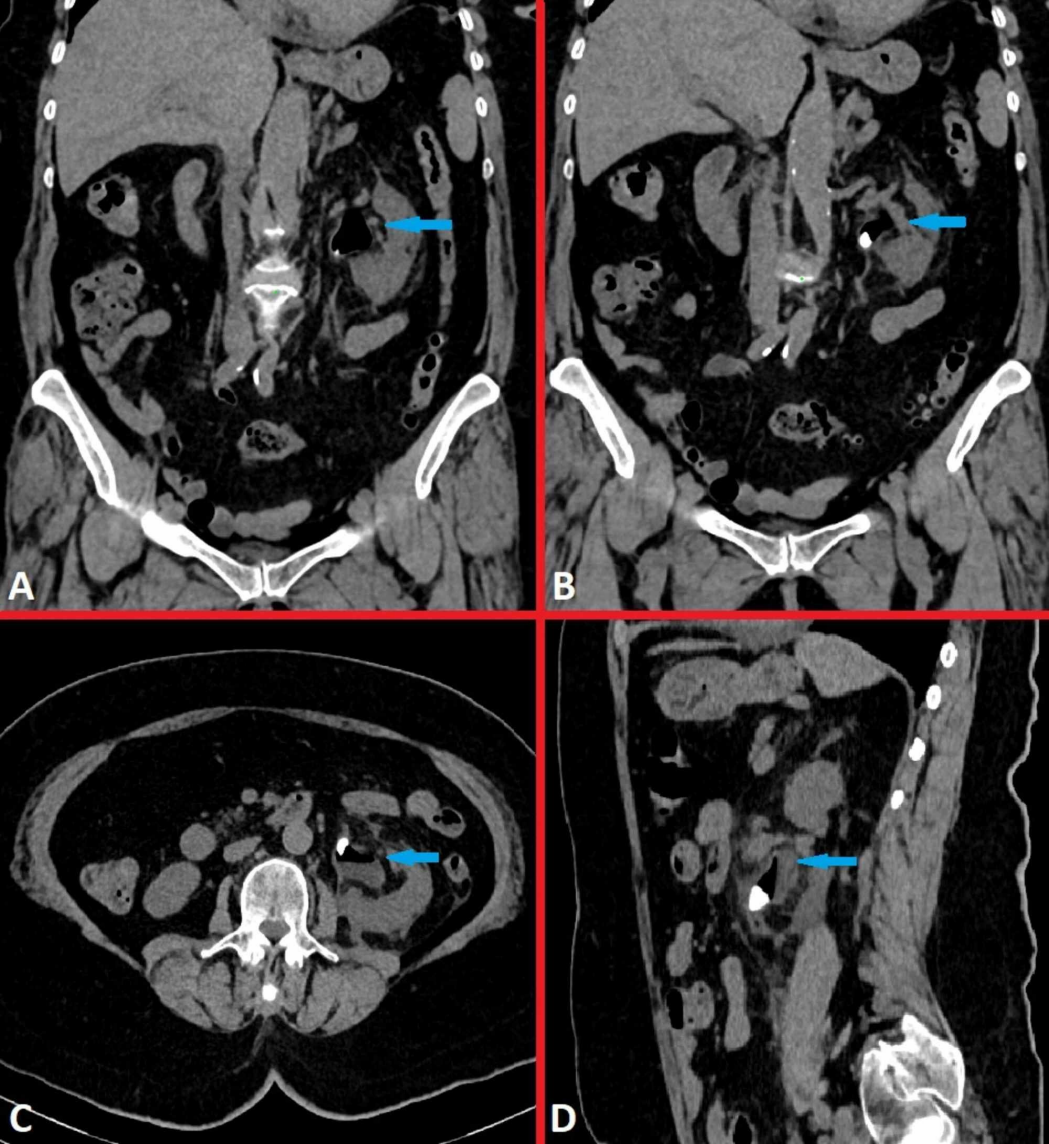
Radiological imaging during admission to the emergency room A 10-mm sized stone and gas image in the left renal pelvis, in coronal (A,B), in transverse (C) and in sagittal cross-sections on NCCT ((D). NCCT: non-contrast computed tomography.
